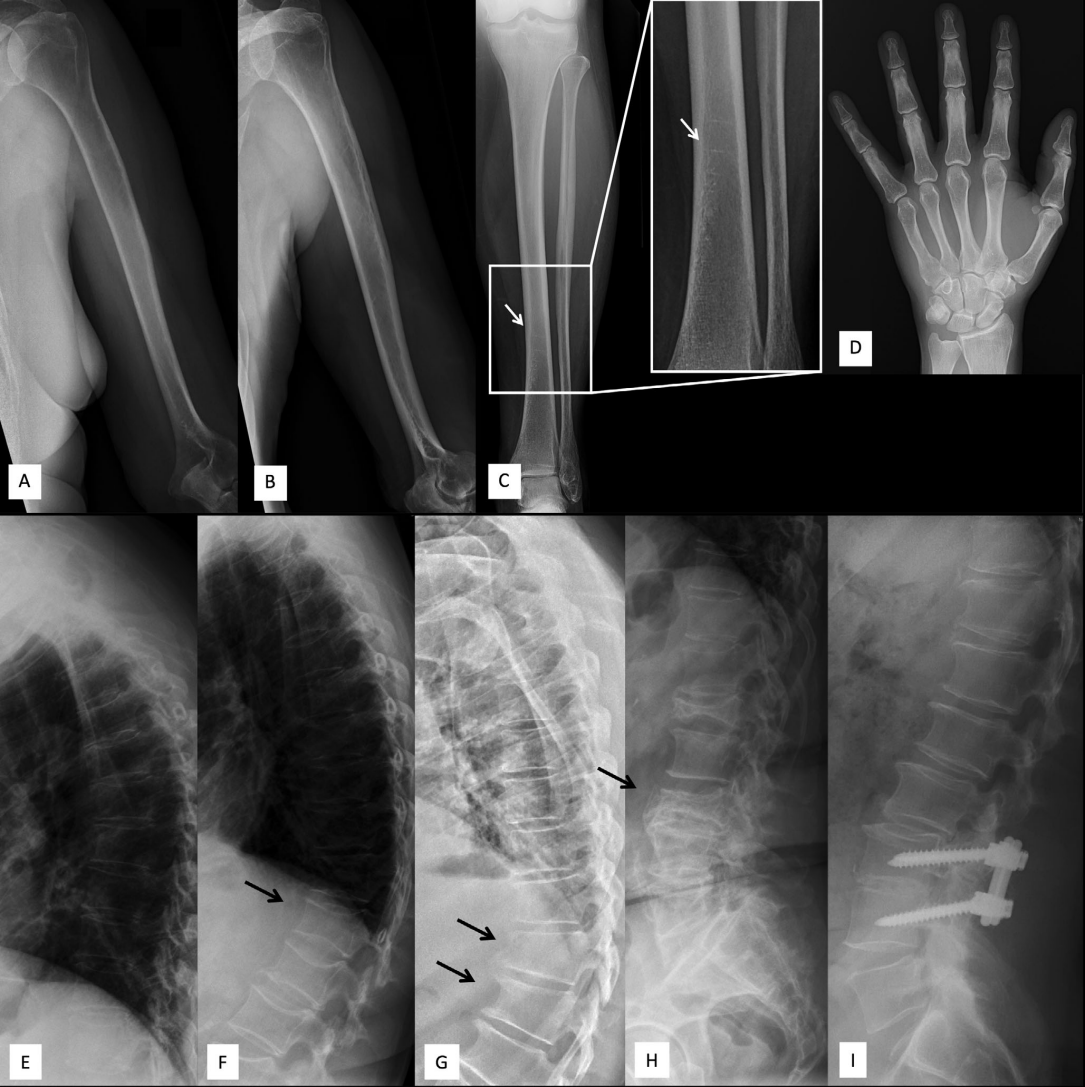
Long bone, hand, and spinal radiographs of four subjects with a heterozygous p.G218R *ARHGAP25* mutation. Images show visible trabeculation and horizontal growth arrest–lines (white arrow) indicating poor mineral content and disturbed bone turnover, and multiple vertebral compression fractures (black arrows), particularly near the thoracolumbar junction of the spine, and subsequent exaggerated thoracic kyphosis and straightened lumbar lordosis. Upper extremity images of (*A*) 69‐year‐old female (II‐2, index) and (*B*) 64‐year‐old female (II‐4). Lower extremity image (*C*) and left hand (*D*) of 69‐year‐old female. Thoracic spine radiographs of (*E*) 69‐year‐old female, (*F*) 64‐year‐old female, and (*G*) 61‐year‐old male (II‐6). Lumbar spine radiographs of (*H*) 69‐year‐old female (II‐2, index), and (*I*) 61‐year‐old male.
